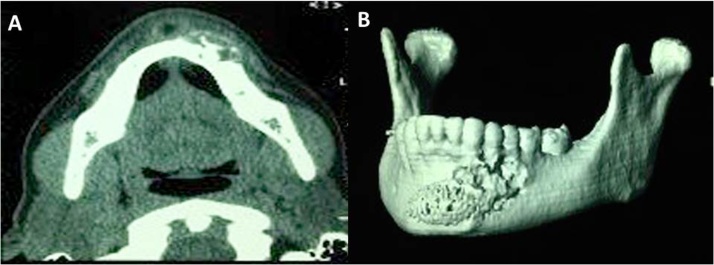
A – Axial cut of the computed tomography showing medullary and cortical bone destruction, not affecting the inner mandibular cortical surface. B – Computed tomography with 3D reconstruction, showing the alloplastic material in the chin region. Because the patient concealed such information, the image identified suggested a bone tumor lesion, either malignant or with aggressive local behavior, of odontogenic or non-odontogenic mandibular origin.
